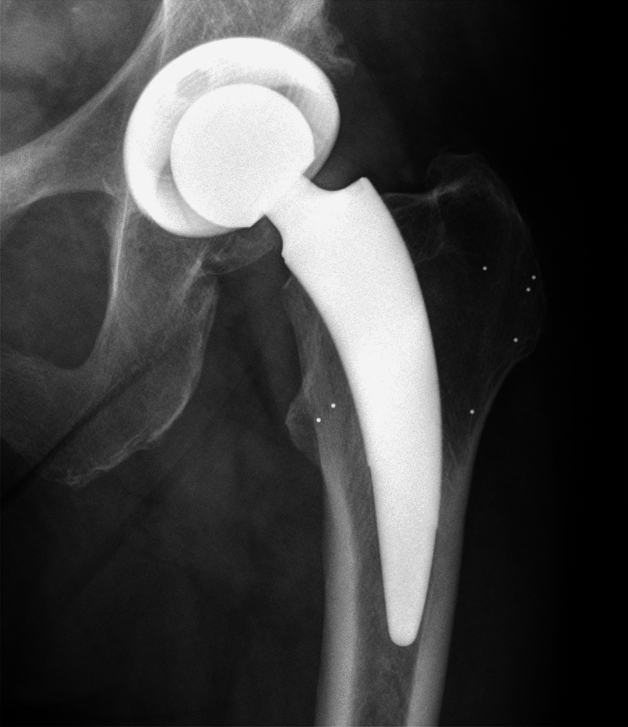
X-ray image of the implant and the surrounding tantalum markers in the trochanter major and minor.
